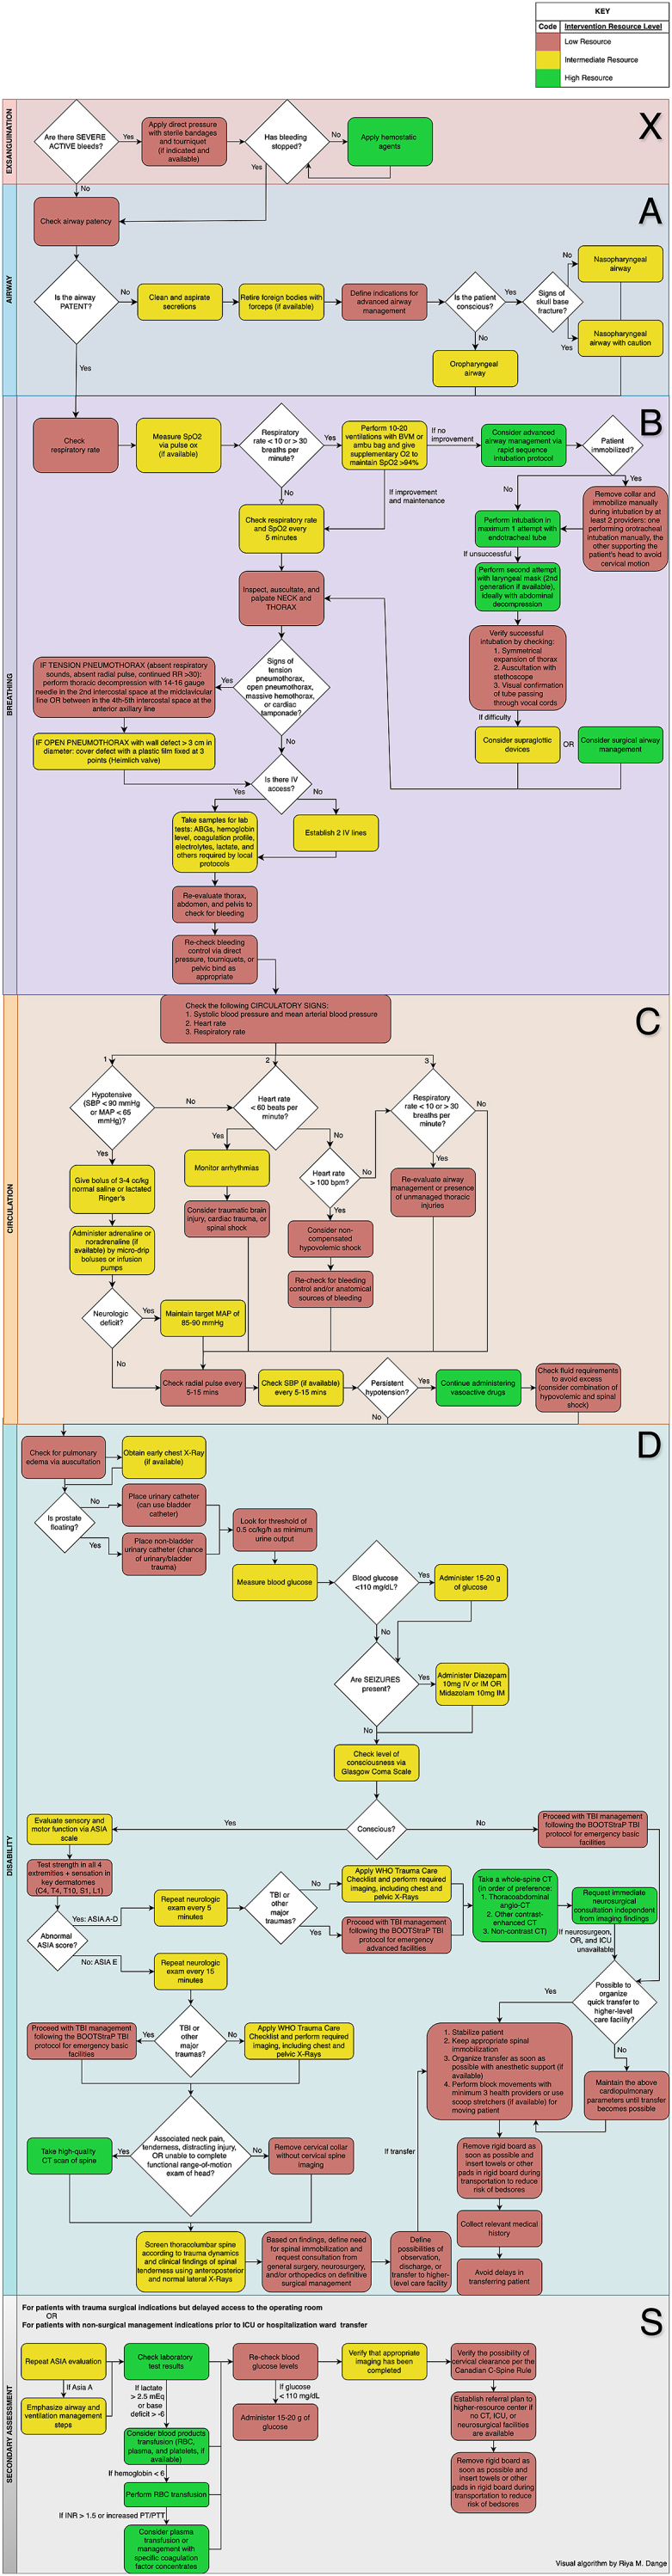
Algorithm for the management of an adult with suspected spinal trauma in a medium-advanced facility (with CT scan).
